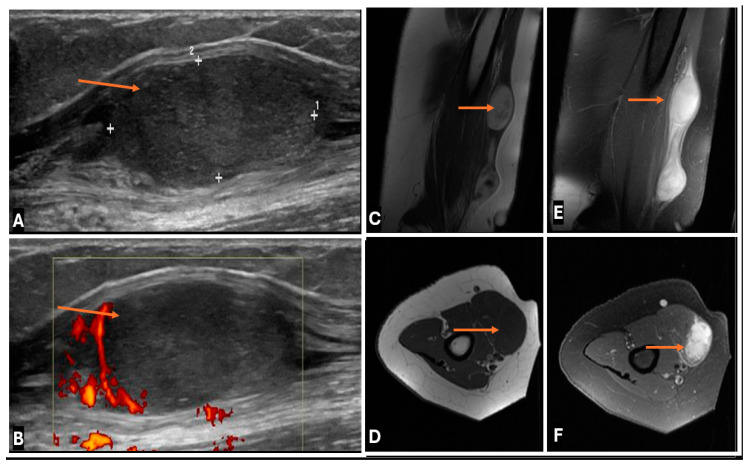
Primary Spindle cell sarcoma of the median nerve in a 32 year old female. (A–F) (A) Gray scale image (B) Color doppler image (C) T2-w Sagittal; (B) T2-w FS Sagittal; (C) T1-w Axial (D) T2-w FS Axial images showing Spindle cell sarcoma of the median nerve in the arm as relatively well circumscribed mass (thin arrows), with low to intermediate T1 signal and high T2 signal on MRI and appears hypoechoic on ultrasound with peripheral vascularity on colour doppler images.
